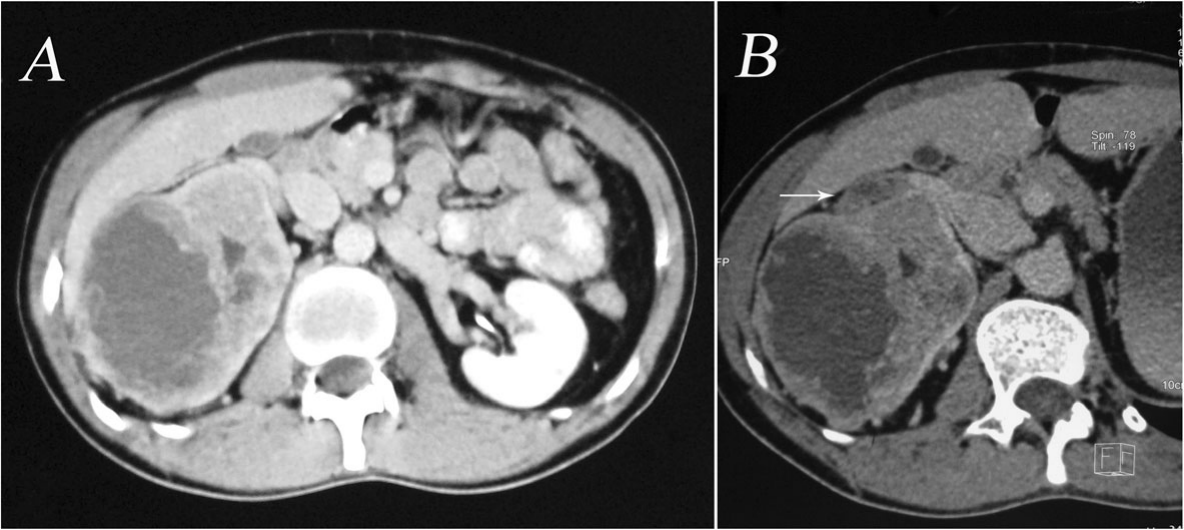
a. CT scan showing an 8.0-cm right-side heterogeneous renal mass infiltrating the renal calices. b. CTA showing a right-side renal tumor with tumor thrombus in the right renal vein (white arrow)
